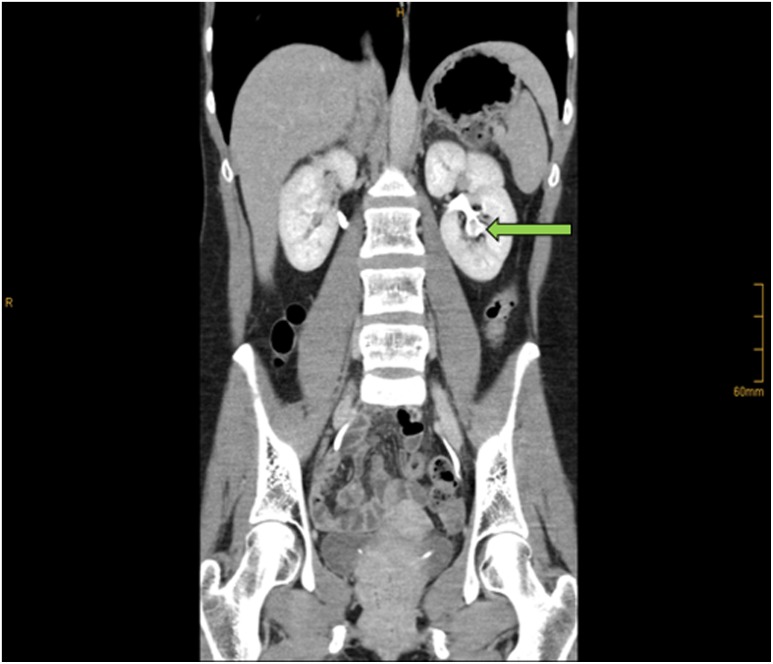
CT urogram showing filling defect in the left lower pole calix.
